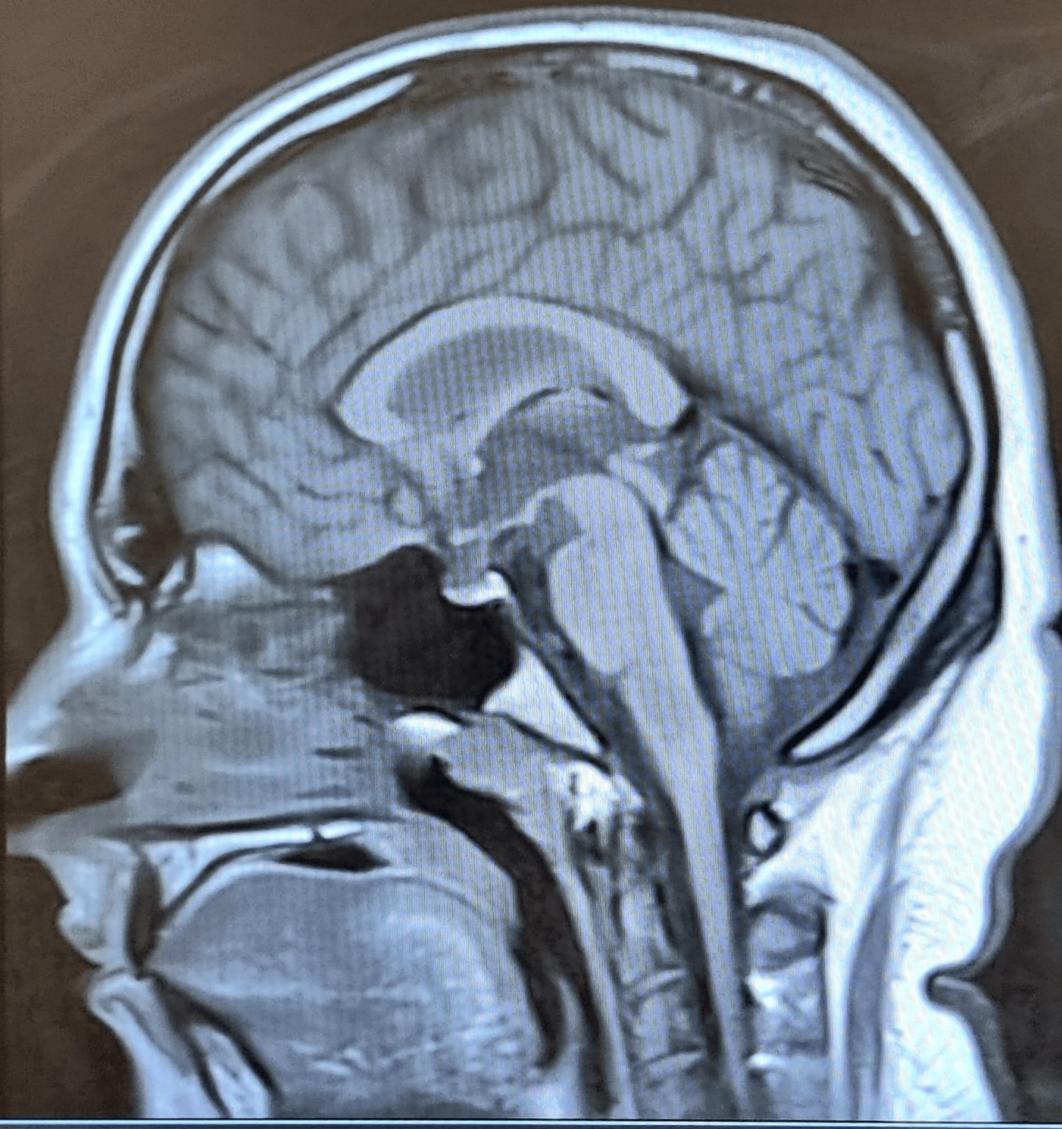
The MRI of Case 3's brain (sagittal T1 image) shows no gross pathology.
